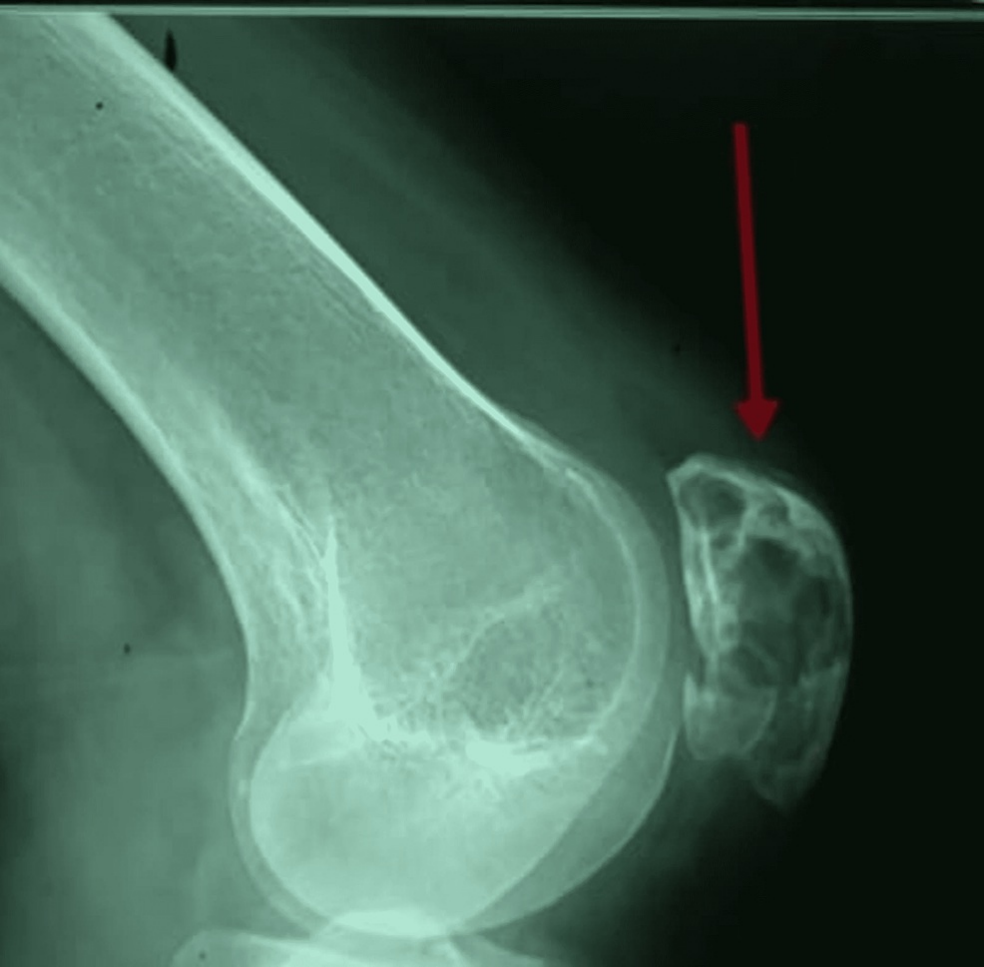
Lateral radiograph of the left knee joint: circumscribed septate osteolytic lesions seen in the patella.
